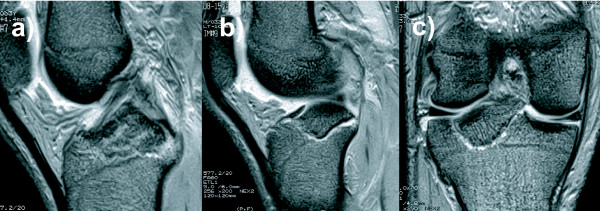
Sagittal and coronal T2-weitghted magnetic resonance images of the knee, showing upward displacement of the PCL insertion at the tibia (a) and the displaced fragment of the lateral tibial condyle (b and c).
